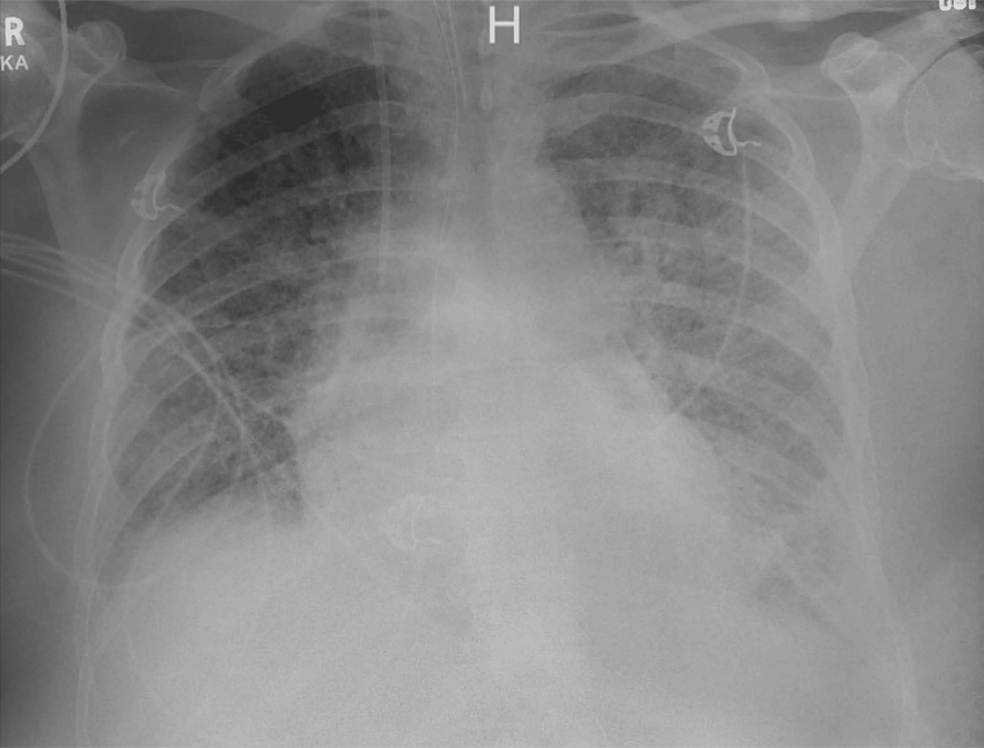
A repeat CXR showing worsening of the diffuse airspace disease bilaterally more on the left field with blunting of the costophrenic angle CXR, chest x-ray
